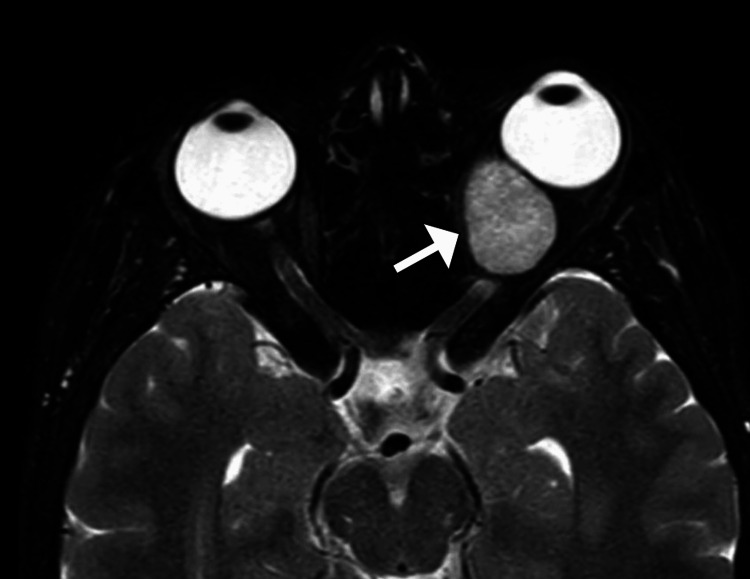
Axial MRI T2-weighted image demonstrating a well-defined, hyperintense intraconal lesion in the left orbit, consistent with a cavernous hemangioma (arrow) The lesion is causing mild proptosis without evidence of optic nerve compression or infiltration of adjacent structures.
